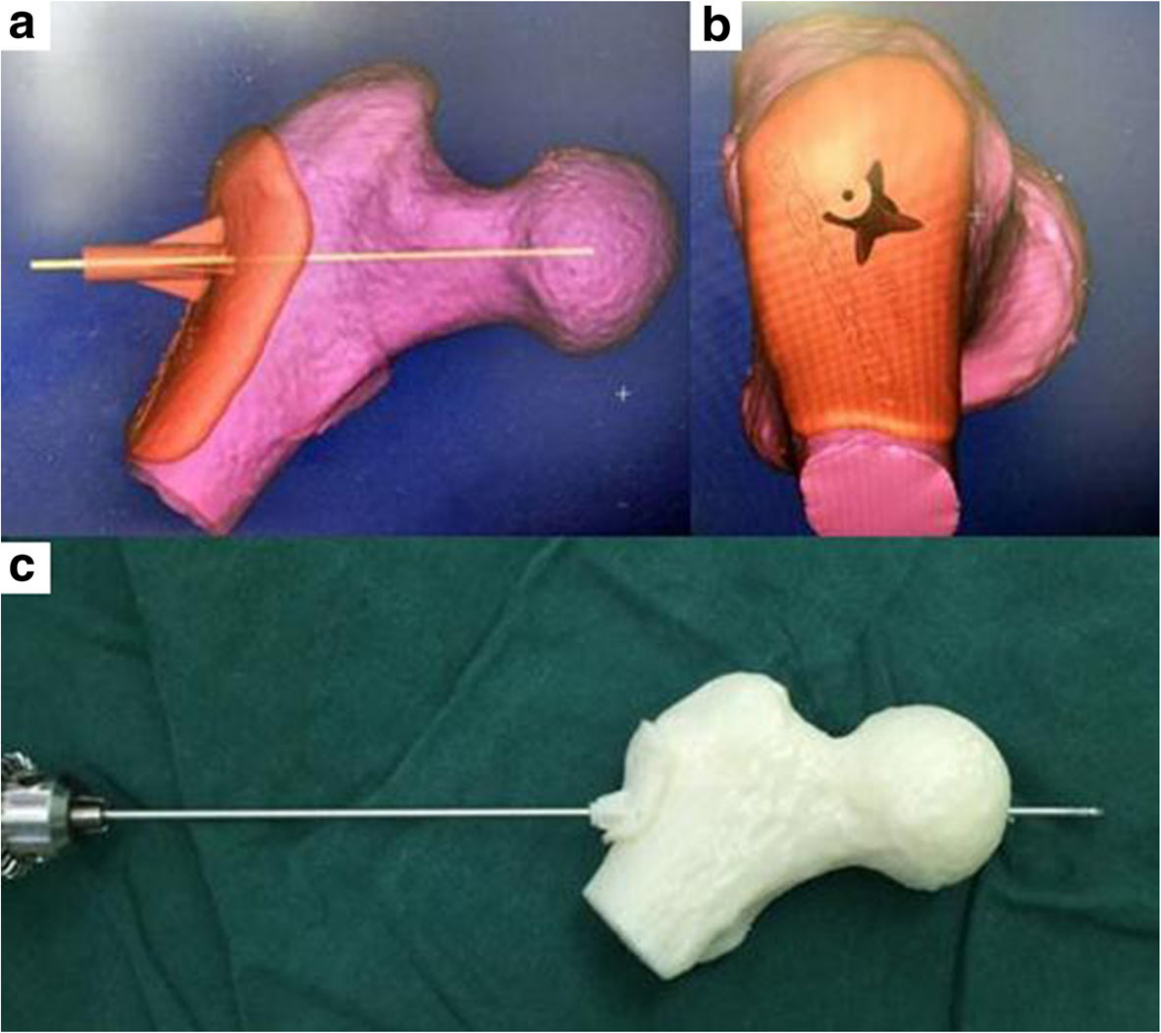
a The pre-operative 3D CT reconstruction images. b A virtual guide pin path was established on the proximal femur model using MedCAD, making the path reach to the target region. c The guide pin path was established with the assistance of the corresponding guide pin guide plate, which was performed at the opposite side by the same method
